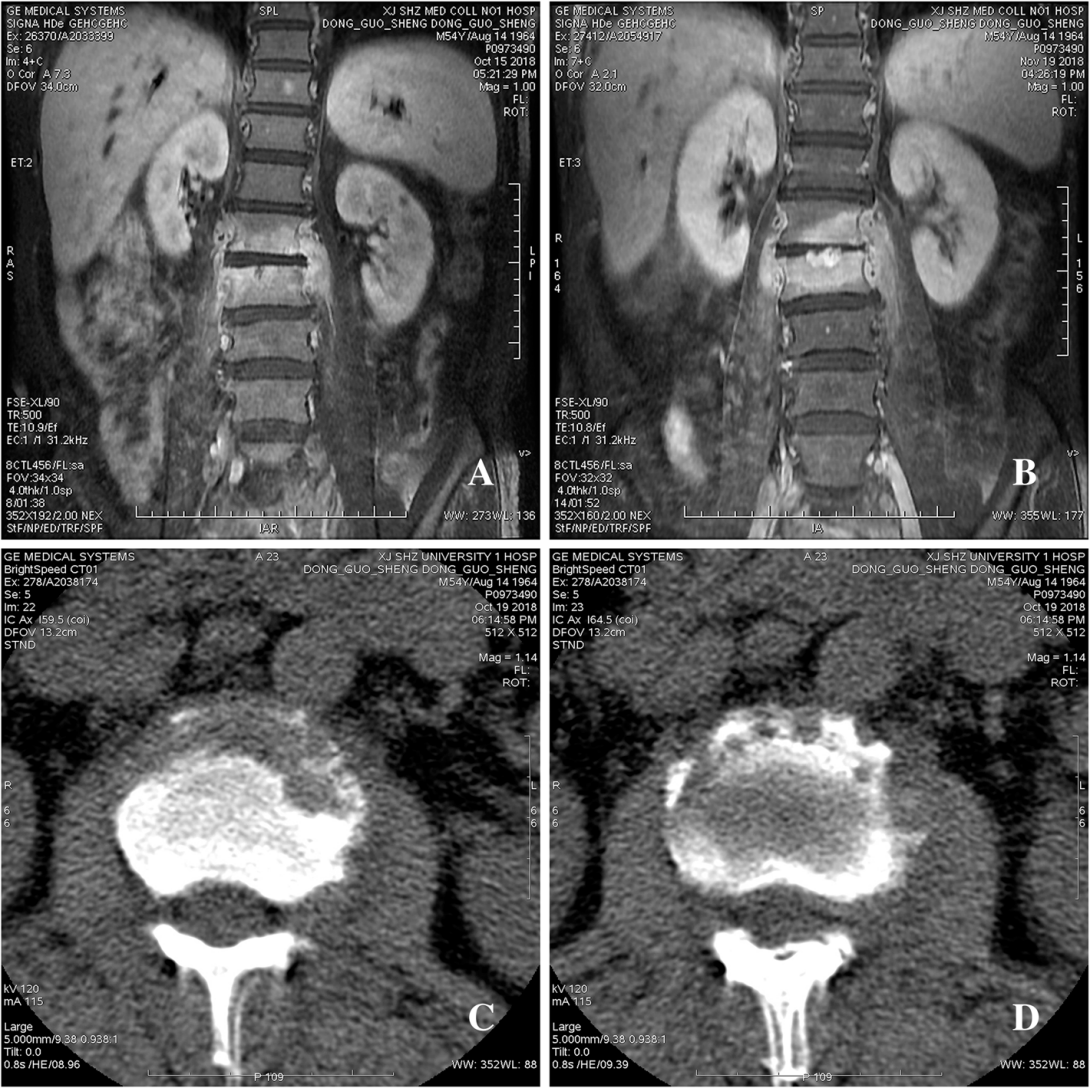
The patient with *Brucella* spondylitis at L23. ad The MRI and CT examinations showed the destruction of the vertebral bodies of L23 at different times. a, b The MRI examination on October 15 and November 19, 2018. c, d The CT examination on October 15 and November 19, 2018. The red arrows indicate abscess-formed area, and the green arrows indicate the hyperplasia-formed area
